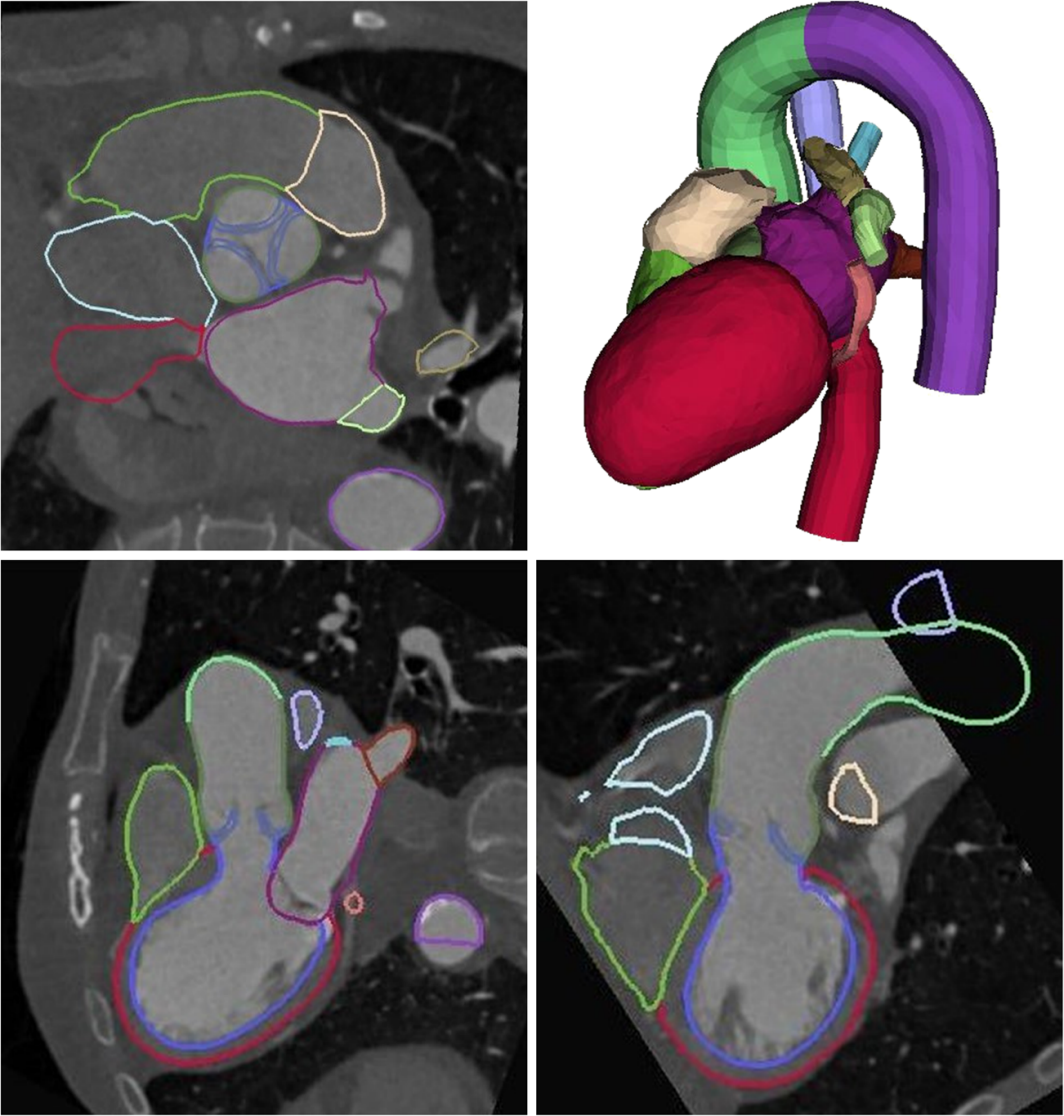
Exemplary visualization of three orthogonal cross sections of CT images featuring an open AV. The colored contours represent the segmentation of the different compartments of the heart. In the upper right panel, the 3D geometry of the segmented compartments is shown
